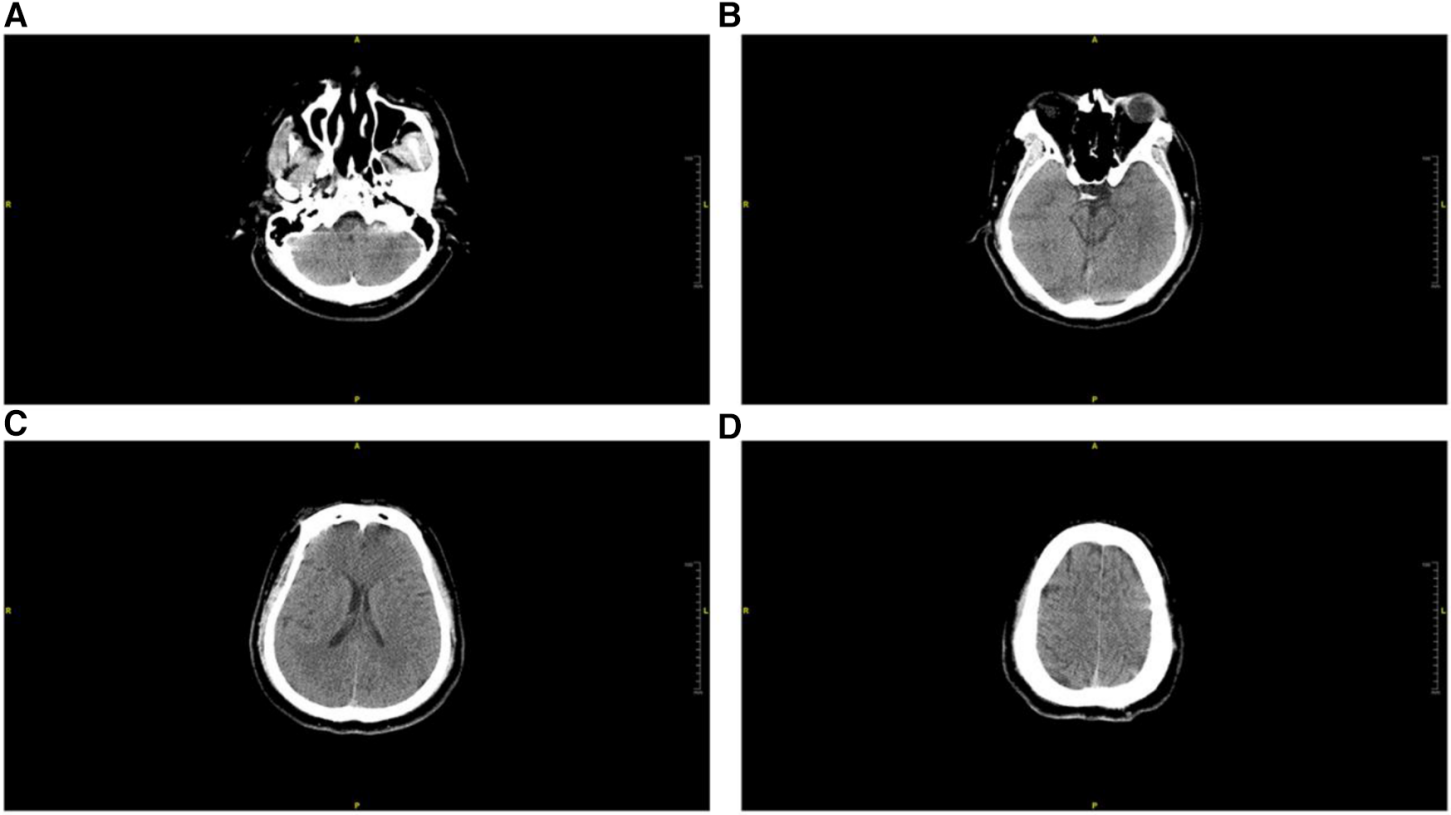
Head CT scan conducted in the emergency department during admission. (A) Cross-sectional view of the petrous part of the temporal bone, (B) cross-sectional view of the pons, (C) coronal section of the radiata, (D) superior section of the frontal lobe. There were no discernible brain lesions observed in any of the examined perspectives.
